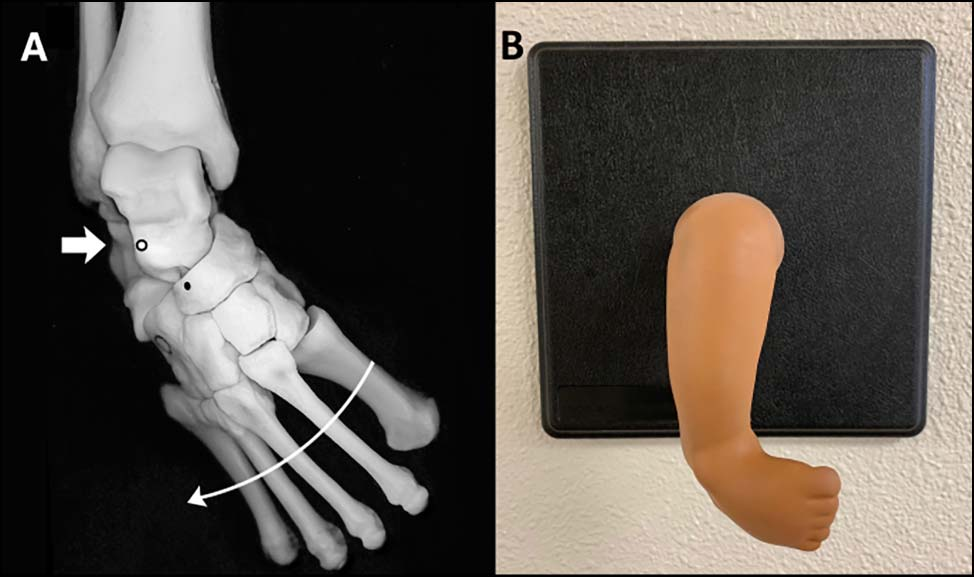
A, Radiograph showing anatomic bone model used to correlate the bony anatomy with soft tissue landmarks and to demonstrate manipulation. The forefoot is abducted (curved arrow) with a fulcrum at the talar head (short arrow). B, Photograph showing a right uncorrected clubfoot foot model used during the Ponseti casting portion of Top Gun. (MD Orthopaedics, Wayland, IA.)
